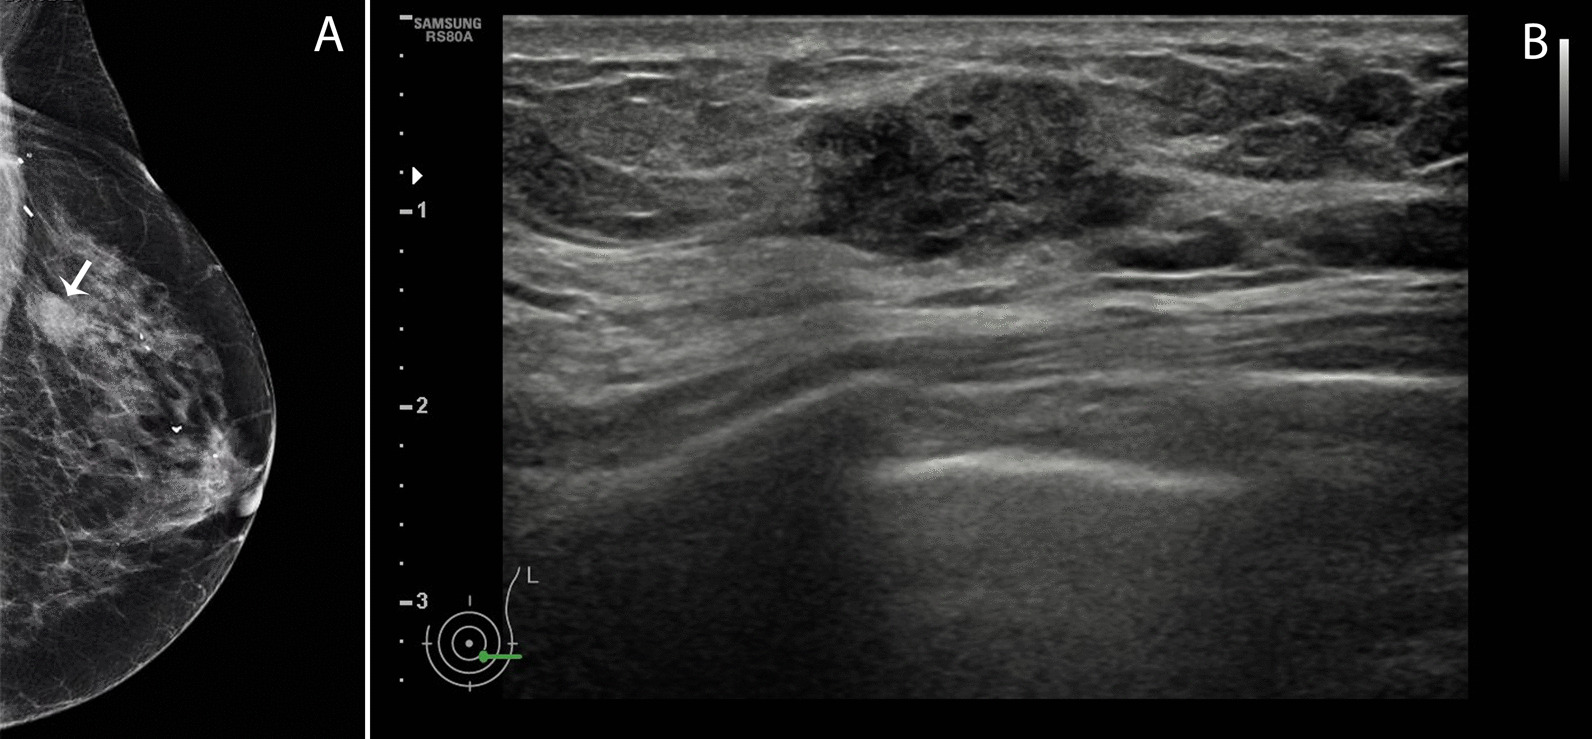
RX and ultrasound imaging of the left breast. A On mammography, the cranio-caudal (CC) prospect shows the presence of a deeply located nodular shaped dense mass at 3 o’clock with irregular borders and highly suspicious of malignancy (white arrow). Sequelae of the previous surgery are visible as well. B On ultrasound the lesion was hypoechoic showing parallel orientation, irregular contours and heterogeneous composition
